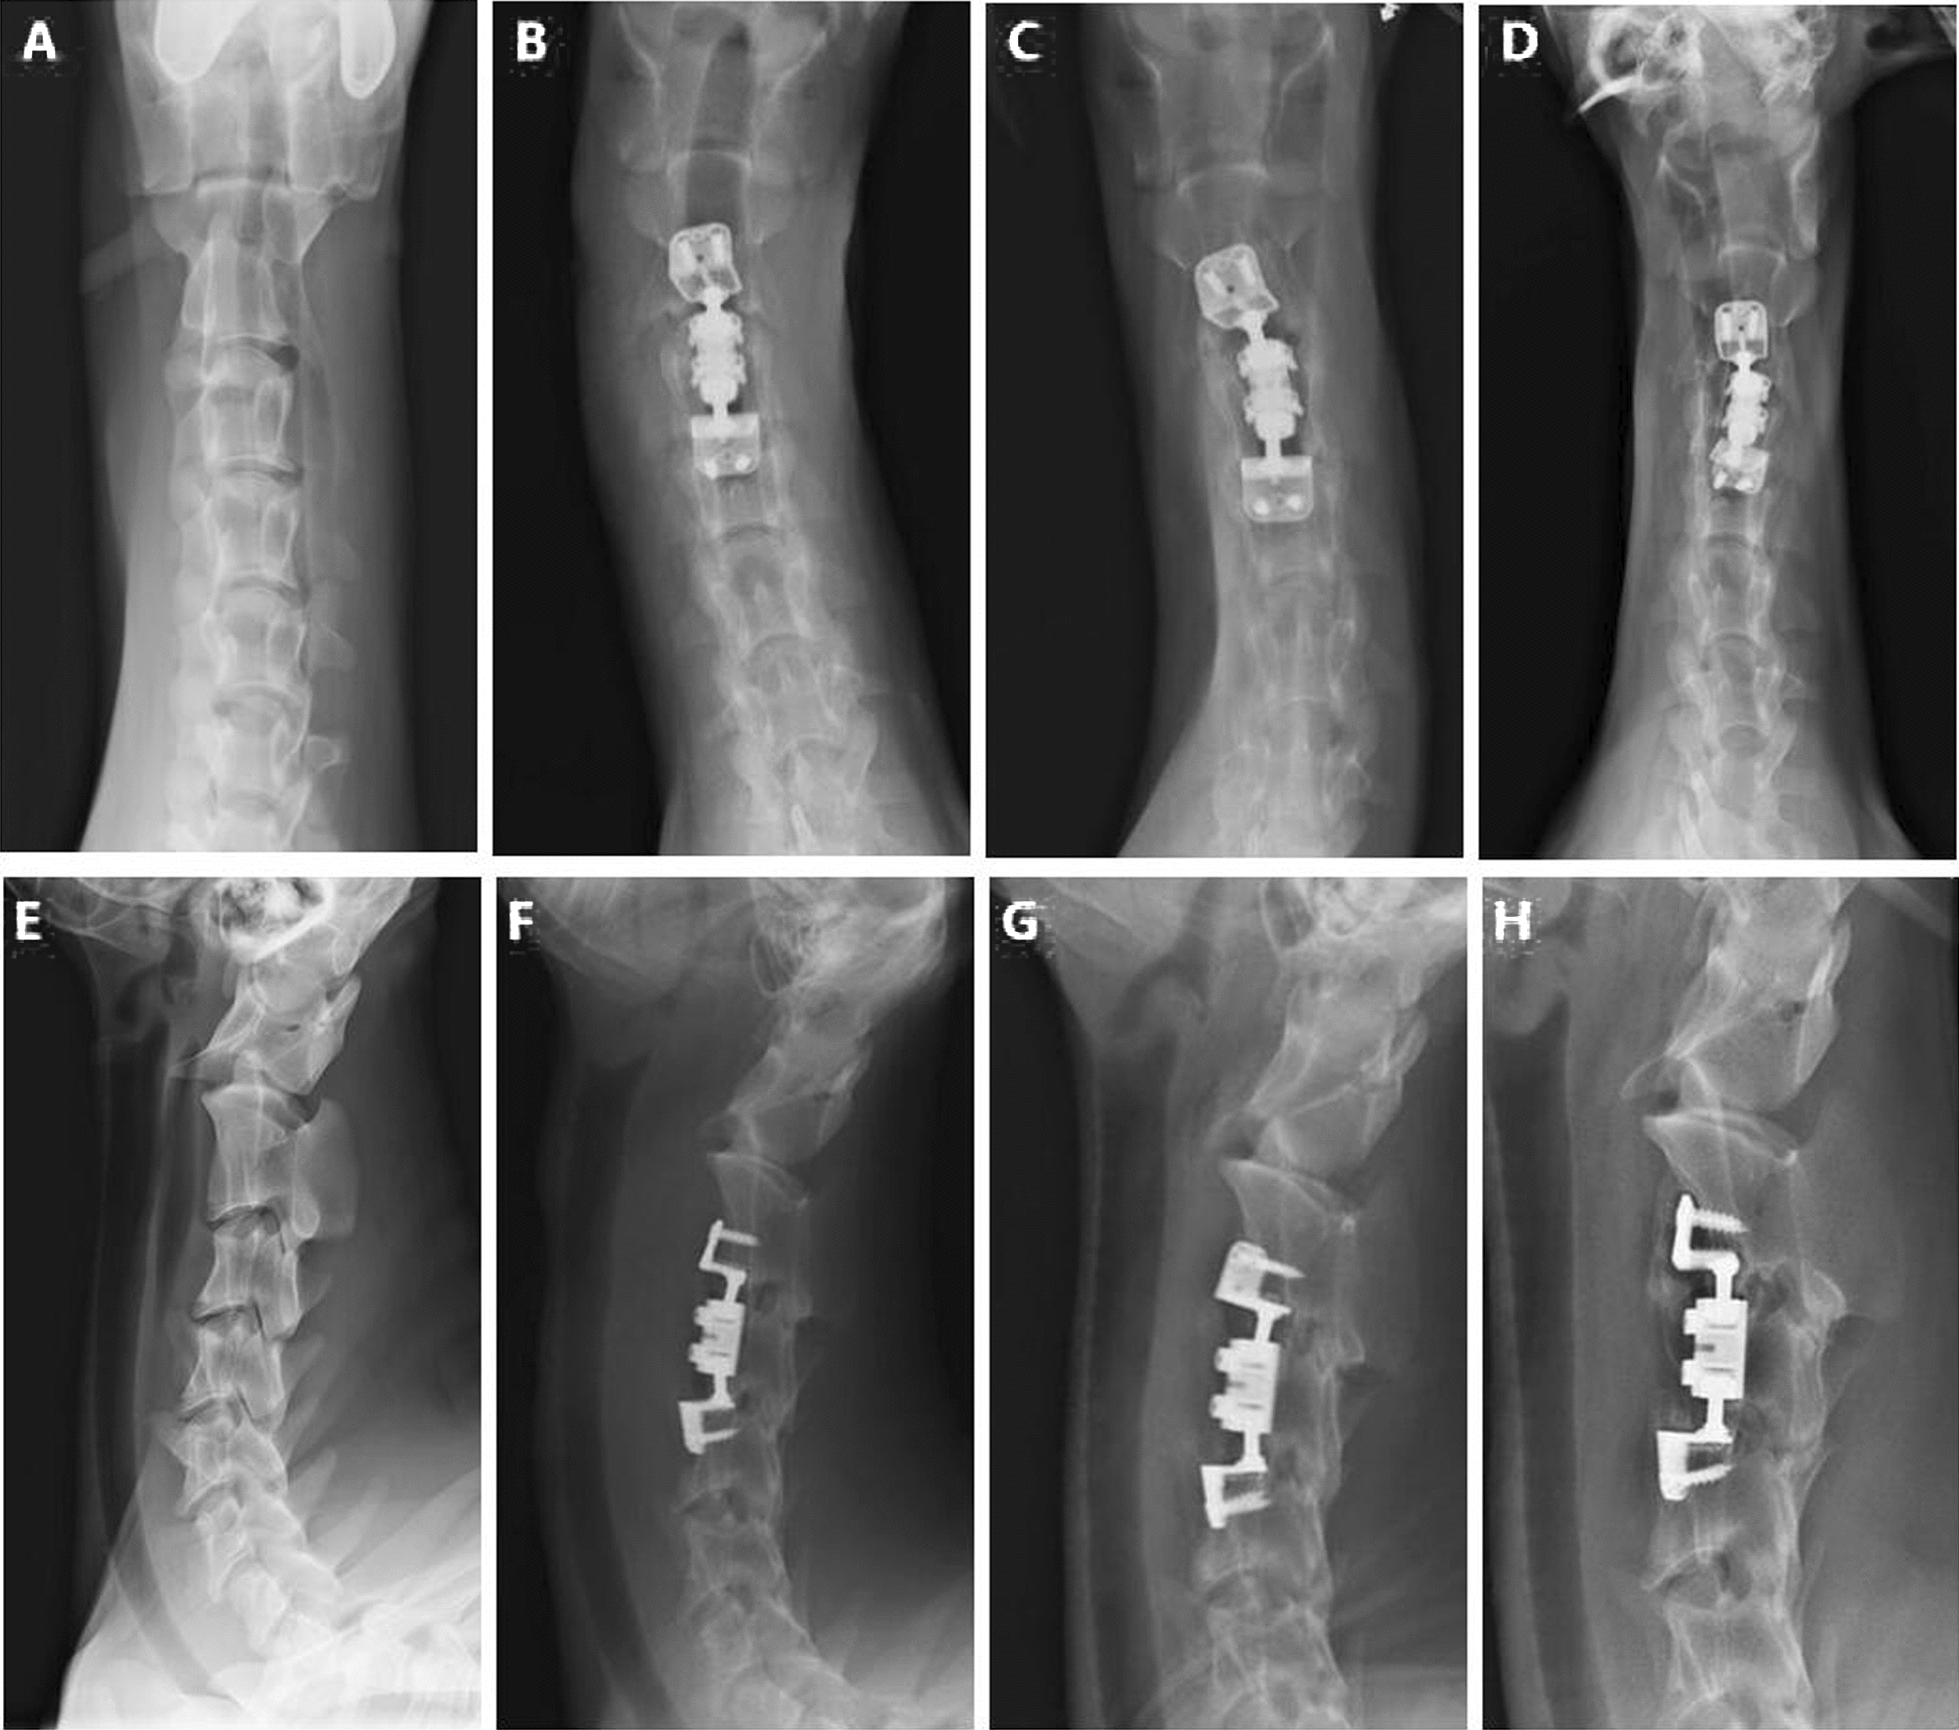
1 Frontal X-ray film. A, pre-operation. B, Postoperative day 1. C, 3months after surgery. D, 6months after surgery. 2 Lateral X-ray film. E, pre-operation. F, Postoperative day 1. G, 3 months after surgery. H, 6 months after surgery
